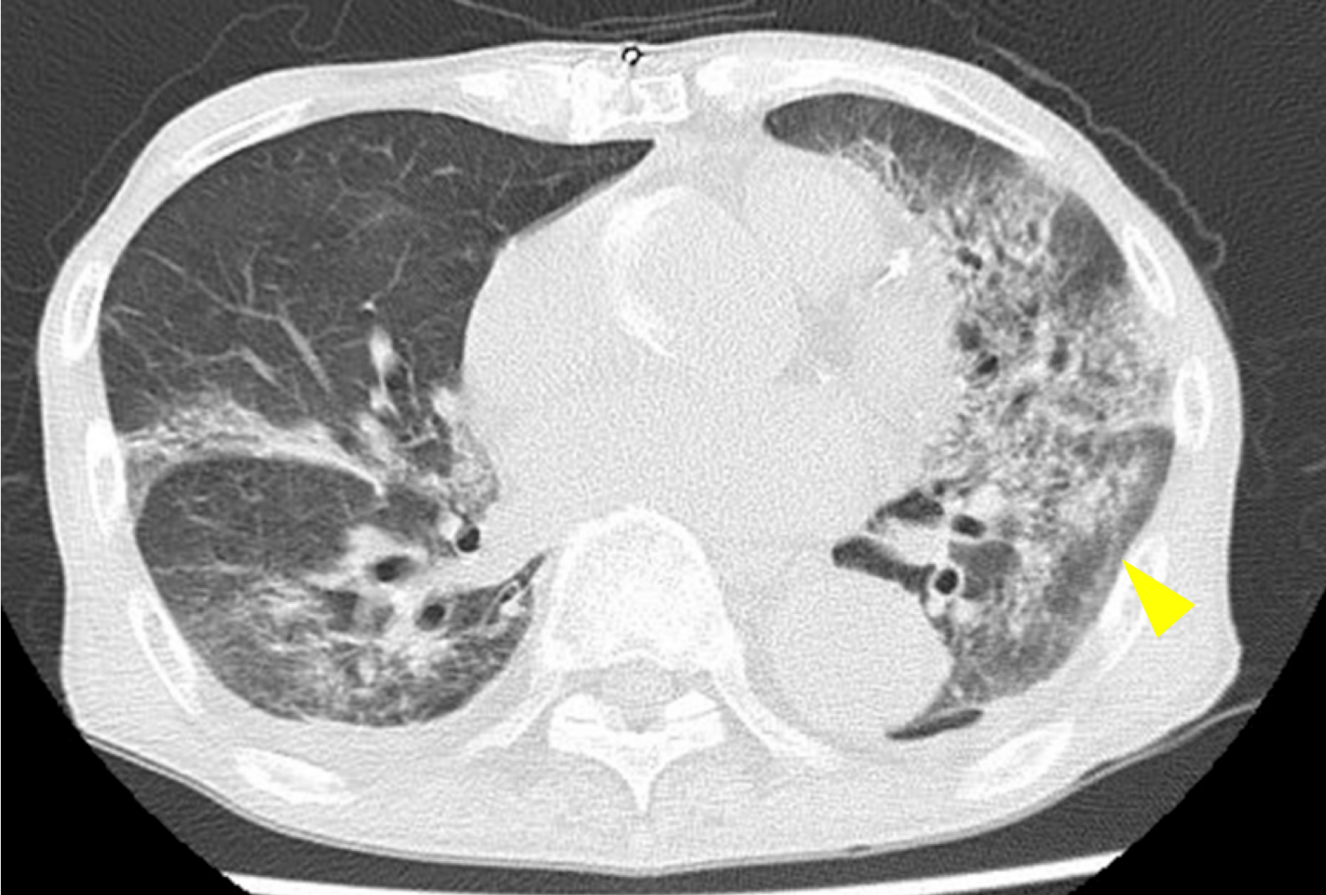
Follow-up chest CT on hospital day five Chest CT demonstrating progression of bilateral ground-glass opacities compared with initial imaging, despite antibiotic therapy for suspected bacterial pneumonia.
